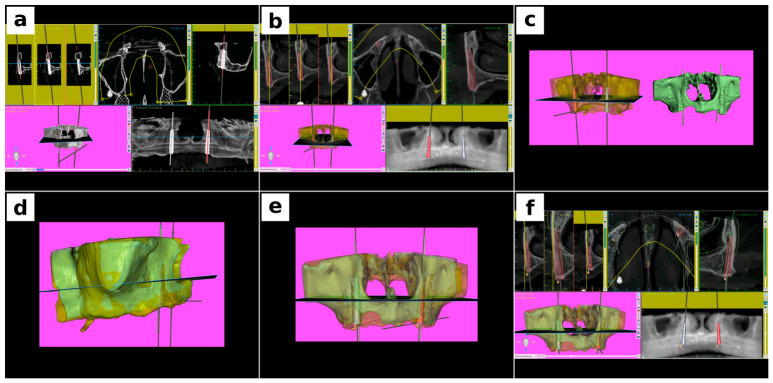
Axis-based verification of implant insertion by superimposition. (a) Study model with metallic implants inserted using the surgical guide. (b) Patient CBCT with virtually planned implants and their axes. (c) Side-by-side visualization of the study model, including the custom axial implants, and the patient’s CBCT dataset prior to alignment. (d) Superimposition of the study model onto the CBCT dataset in the lateral view. (e) Superimposition in frontal view. (f) Superimposition in sectional views, demonstrating minimal deviation between the planned implant axes and the corresponding custom implants.
